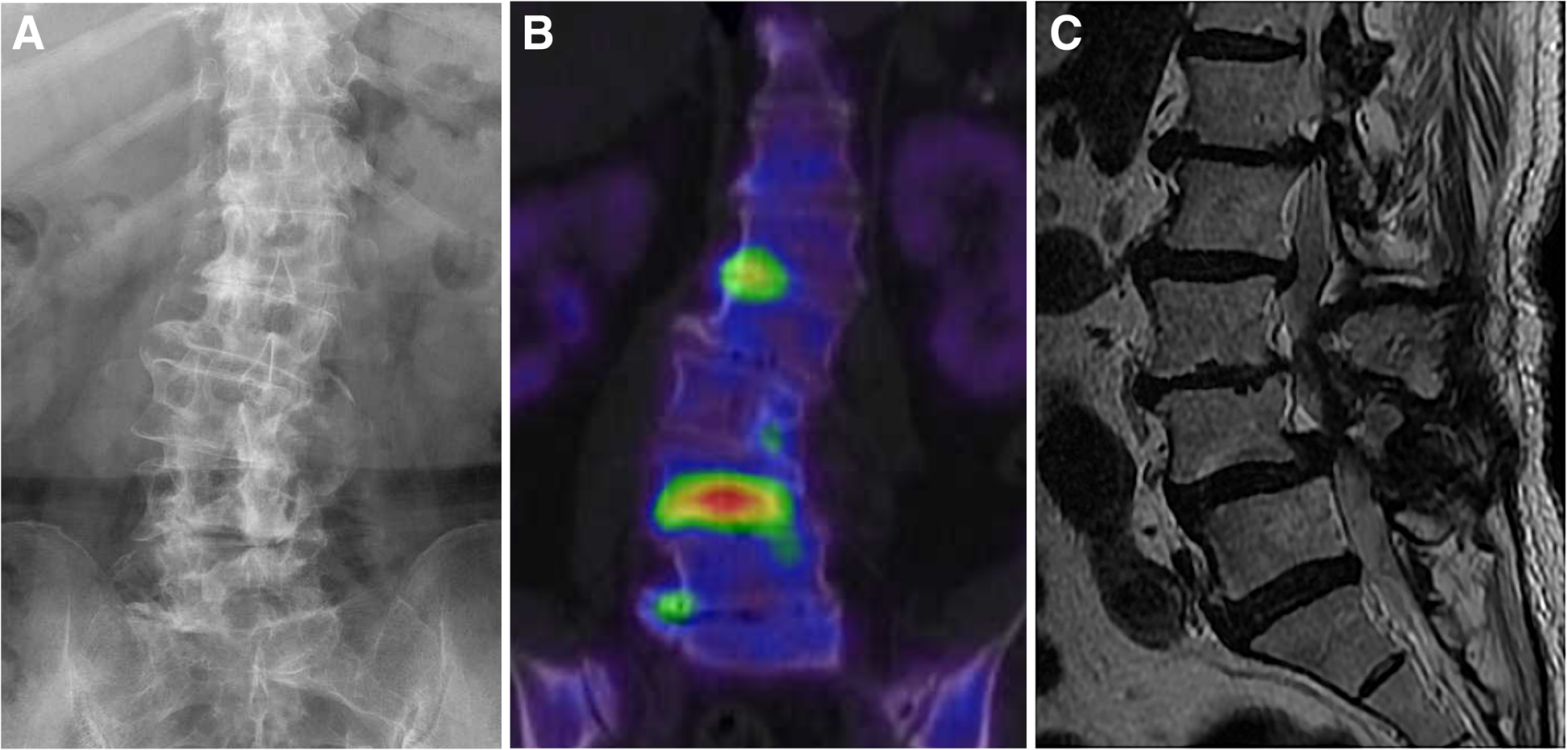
A 74-year-old woman with an insufficiency endplate fracture of L4 vertebral body. a Anterolateral view radiograph showing a degenerative lumbar scoliosis. b Bone SPECT-CT image of the lumbar spine showing a hotspot at the lower endplate of the L4 vertebral body. c T2-weighted MR sagittal image of the lumbar spine without remarkable findings indicating the site of pain-causing pathology. MR, magnetic resonance; SPECT/CT, bone scintigraphy with single photon emission computed tomography
